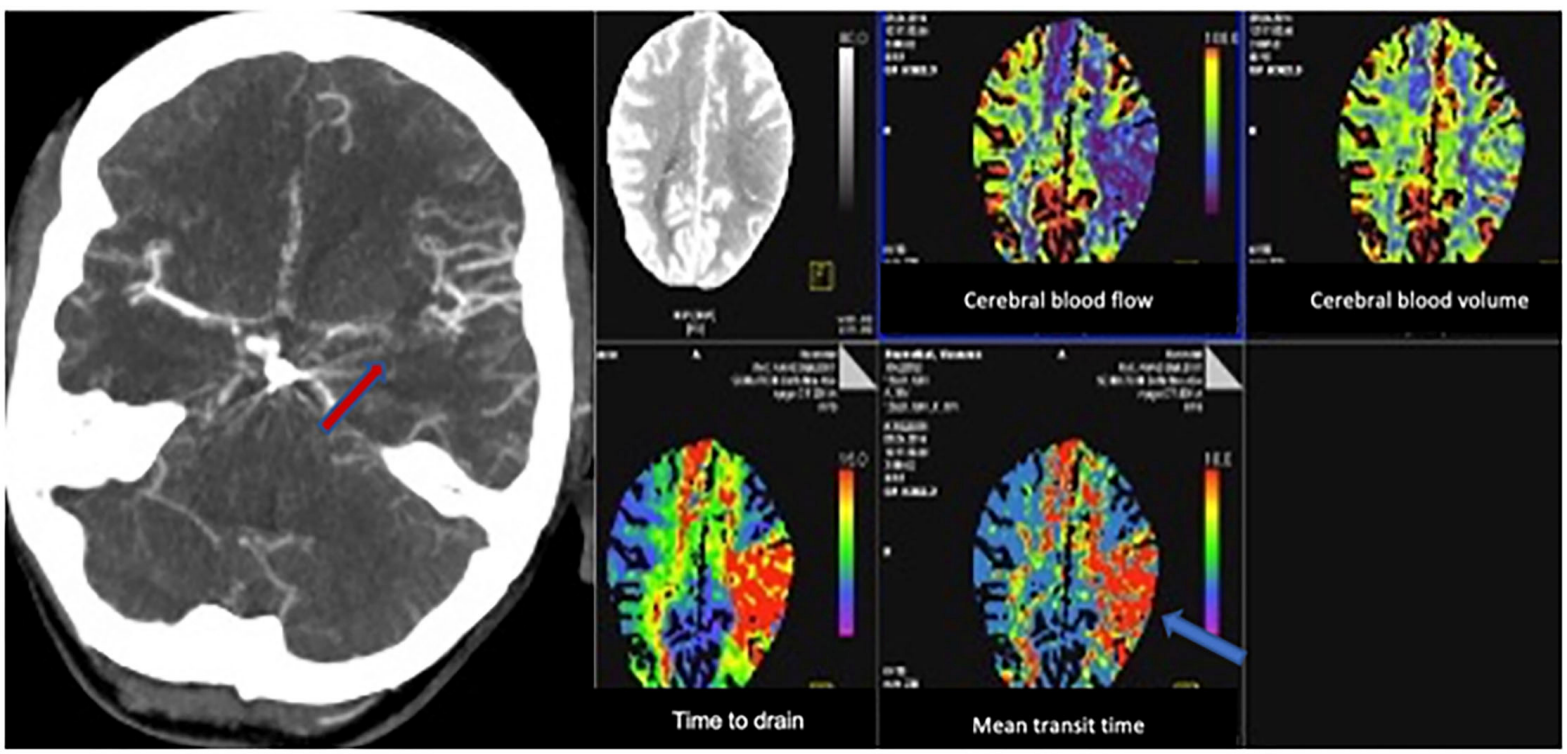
Example of a patient with an indication for ERT with severe vasospasm on the CTA (red arrow, left) with perfusion deficits in the middle cerebral artery territory on the CTP (blue arrow, right).
